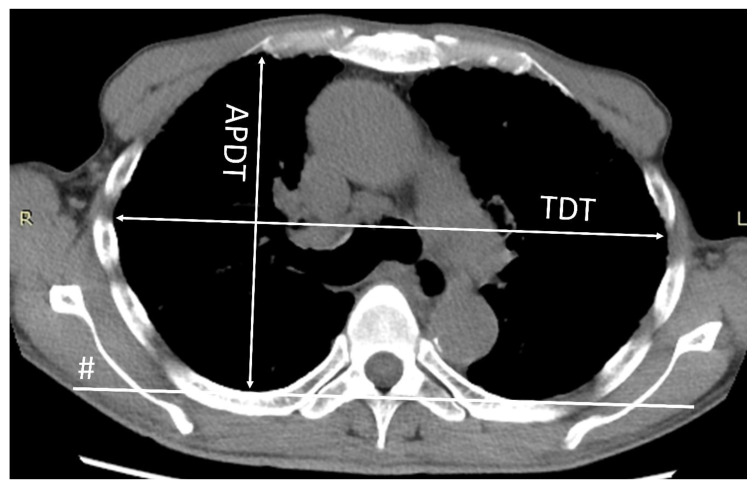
The transverse diameter of the thoracic cage (TDT) was determined as the longest transverse diameter of the thoracic cage measured parallel to a line (#) that runs along the rearmost points of the bilateral 6th ribs in the horizontal section of the CT scan. The anteroposterior diameter of the thoracic cage (APDT) was determined as the longest distance of the anteroposterior dimension of the thoracic cage measured perpendicular to the line along the rearmost points of the sixth thoracic vertebra (line #).
